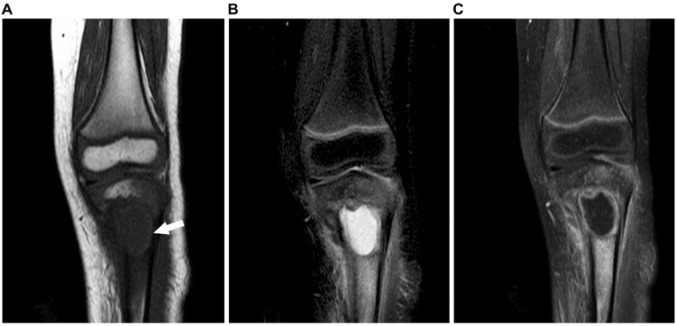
Magnetic resonance imaging findings. (A) The T1-weighted image revealed the penumbra sign (white arrow). (B) The fat-suppressed T2-weighted image revealed very high signal intensity of the central part of the lesion. (C) The gadolinium-enhanced fat-suppressed T1-weighted image showed peripheral rim enhancement.
